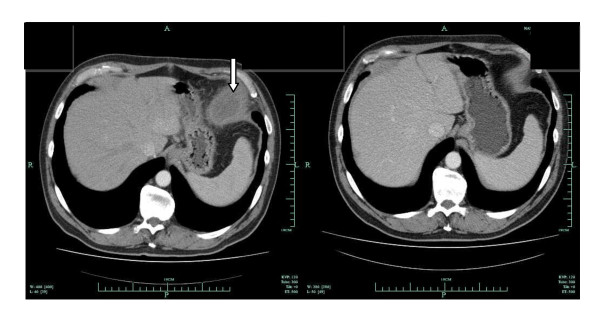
Thoracic computed tomography scan in case two showing a left juxtadiaphragmatic effusion (arrow) which almost disappeared after two weeks of antituberculous therapy.
